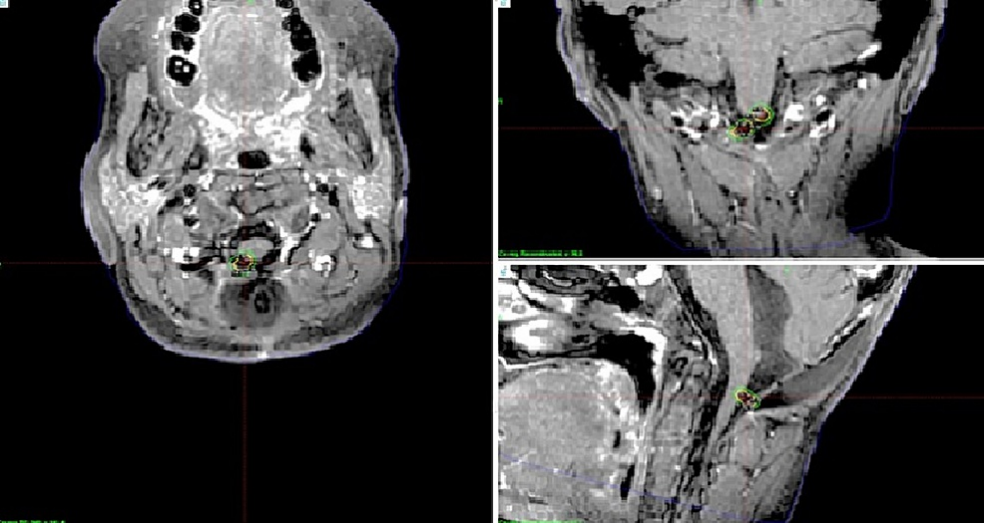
Case 1 Magnetic resonance images of a nine-year-old girl with recurrent ependymoma. Two disseminated small lesions were treated with the prescribed dose of 14 Gy at the tumor margin. Irradiation took 31.5 minutes.
